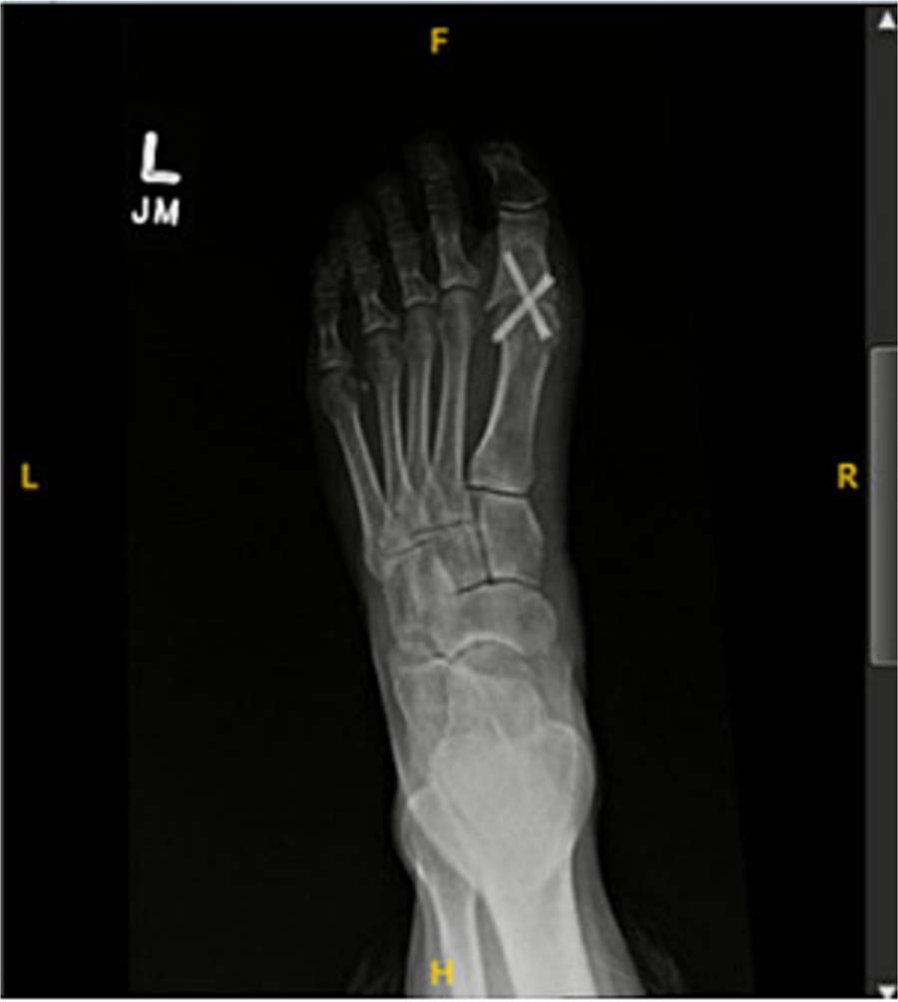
Postoperative radiograph showing first metatarsophalangeal joint arthrodesis with two headless compression screws.
